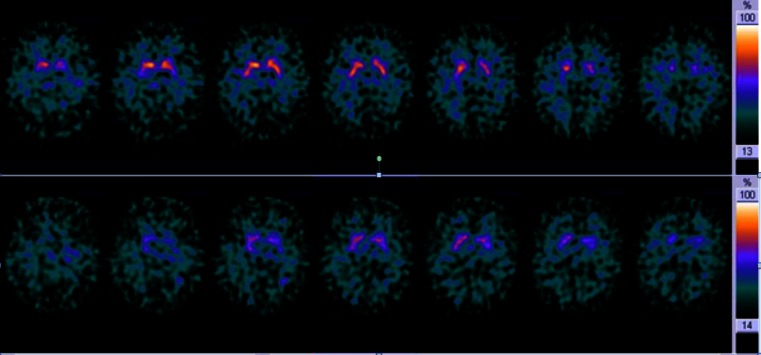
Upper panel*: [^123^I]FP-CIT SPECT scan (transverse slices) acquired in 2011 (binding ratios: right caudate nucleus 2.62, left caudate nucleus 2.56, right putamen 2.37, and left putamen 2.21). *Lower panel*: follow-up [^123^I]FP-CIT SPECT scan acquired in 2012 shows lower tracer binding (binding ratios: 1.82, 1.84, 1.95, and 1.70, respectively)
